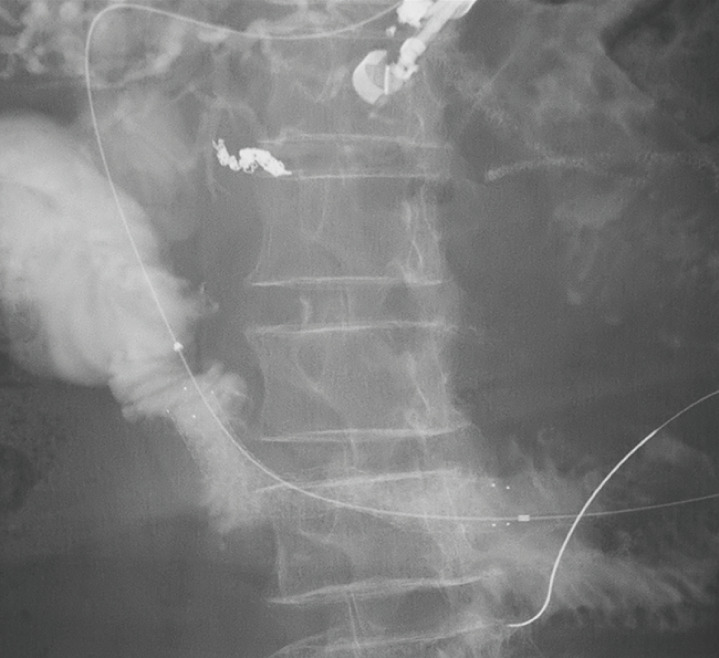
Successful uncovered self-expanding metal stent placement with ultrasound-guided antegrade endoscopic treatment for malignant afferent loop syndrome after biliary reconstruction for cholangiocarcinoma.
